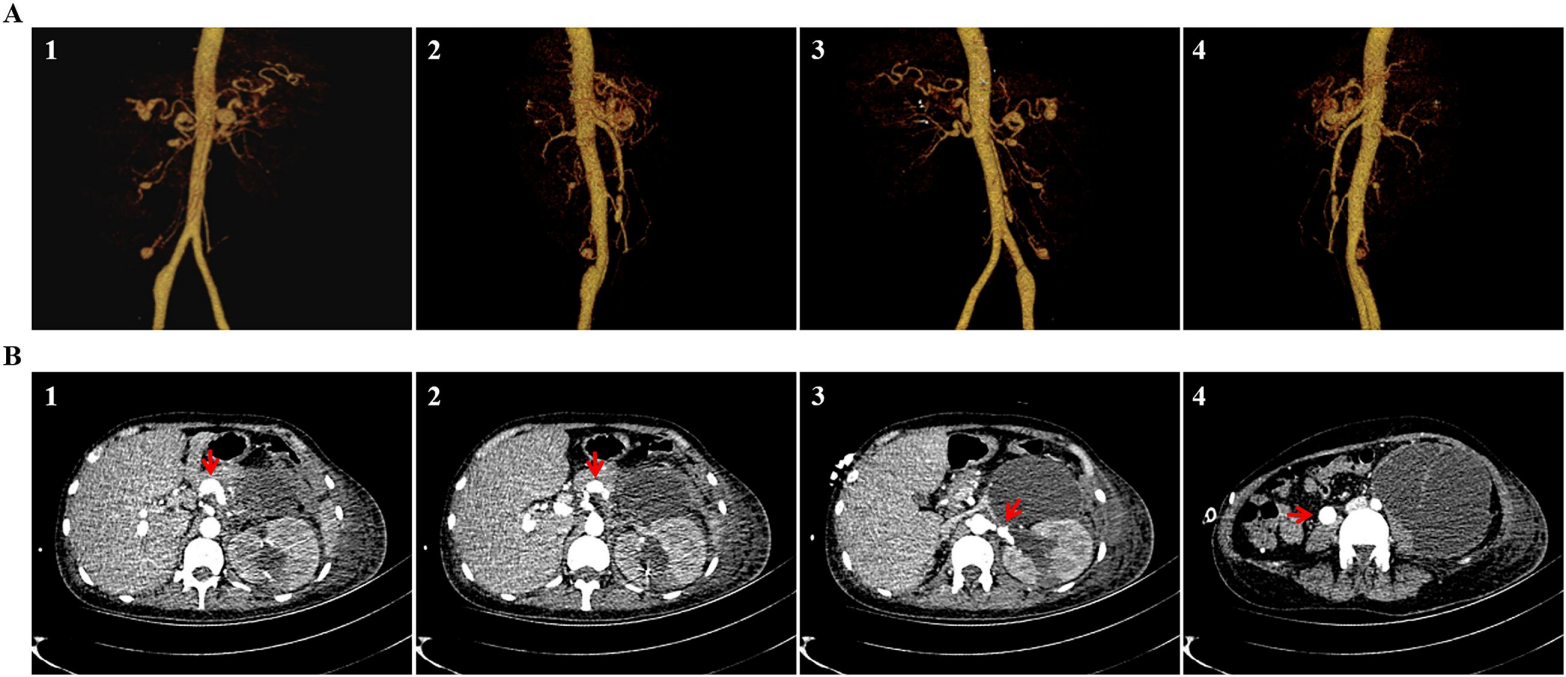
Fibromuscular dysplasia caused recurrent hematoma in the abdominal cavity. A CT angiography indicated an enlarged hematoma on the left of the perinephric space with cystic components and internal septa but no active bleeding site. Fibromuscular dysplasia resulted in stenosis, aneurysm, dissection, occlusion, or arterial tortuosity in the celiac trunk, splenic, bilateral renal, and superior mesenteric arteries. The three-dimensional CT angiographic images are in the anterior (A1), right (A2), posterior (A3), and left (A4) views. The cross-sectional CT images indicate a giant hematoma around the left kidney, aneurysm in the celiac artery (B1), celiac artery stenosis (B2), aneurysm and stenosis in the left renal artery (B3), and aneurysm in the right common celiac artery (B4) (indicated by arrows).
